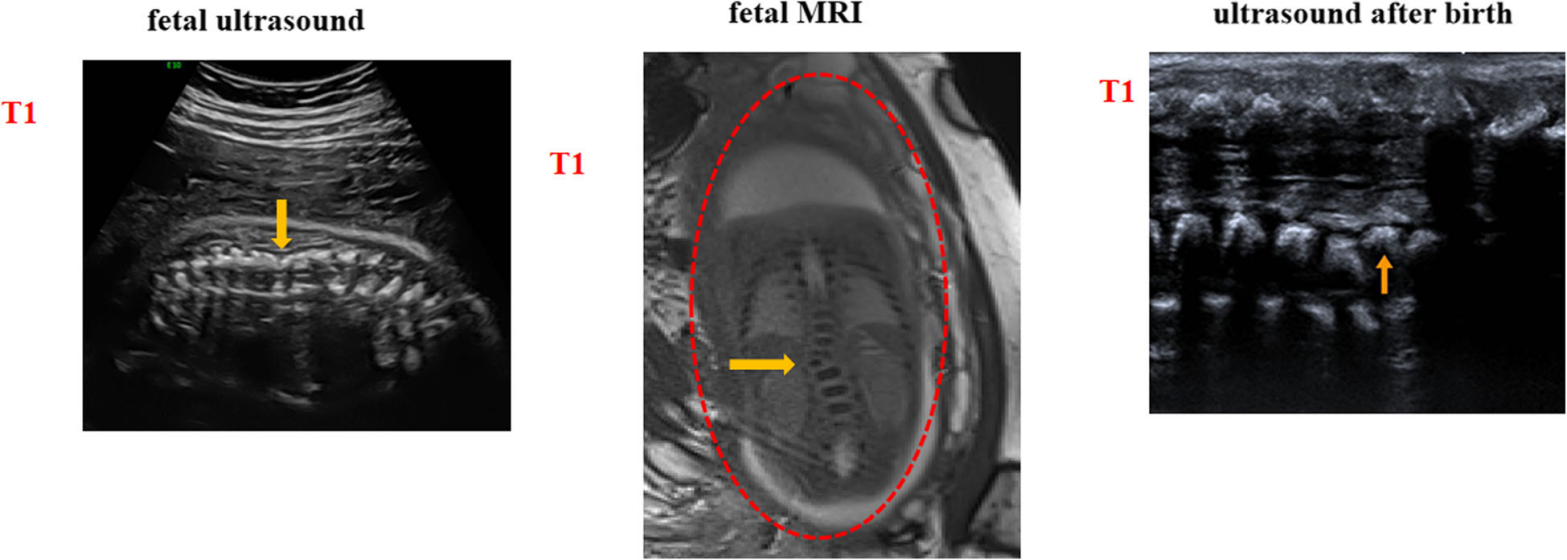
Imaging features of the DCDA twins in case two. Panel 1 shows the fetal ultrasound of the congenital hemivertebrae twin (T1), panel 2 shows the fetal MRI of T1, and panel 3 shows the ultrasound after the birth of T1. The yellow arrow indicates the defect of fetal hemivertebrae, and the dotted red line marks the area indicating the gravid uterus in the fetal MRI. T1 is short for the congenital hemivertebrae twin in case two
